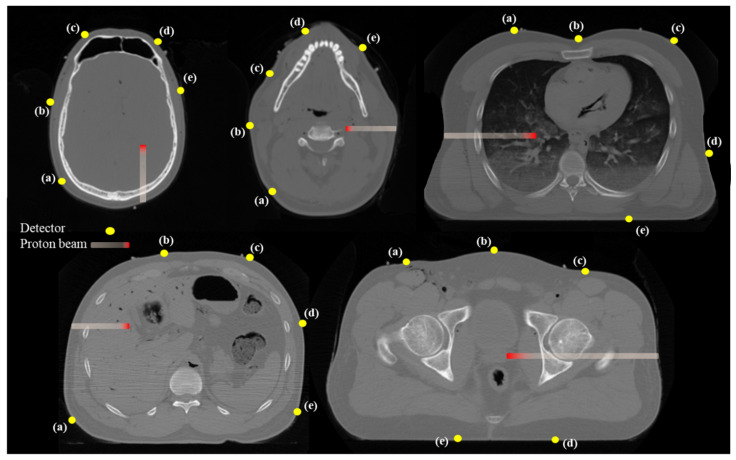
Axial CT images showing the beam path (red) and detector positions (yellow dots) for five clinical sites used to evaluate detector placement adequacy. Each letter (a–e) indicates the position of the detector. Detectors were placed around each target in positions that did not interfere with the proton beam path to avoid perturbation of dose delivery while ensuring adequate acoustic signal reception.
